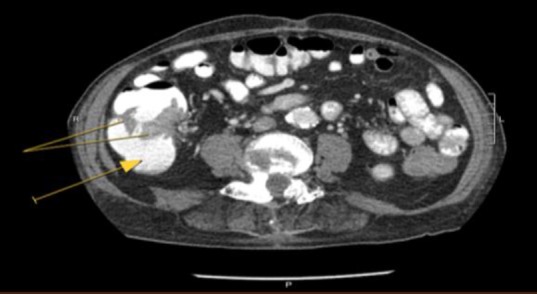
Axial view, CT scan abdomen with contrast: Solid arrow pointing at cecum. Solid lines pointing at contrast enhanced malignant mass. The mass is seen to be encircling more than 80 % of the circumference of the gut wall
